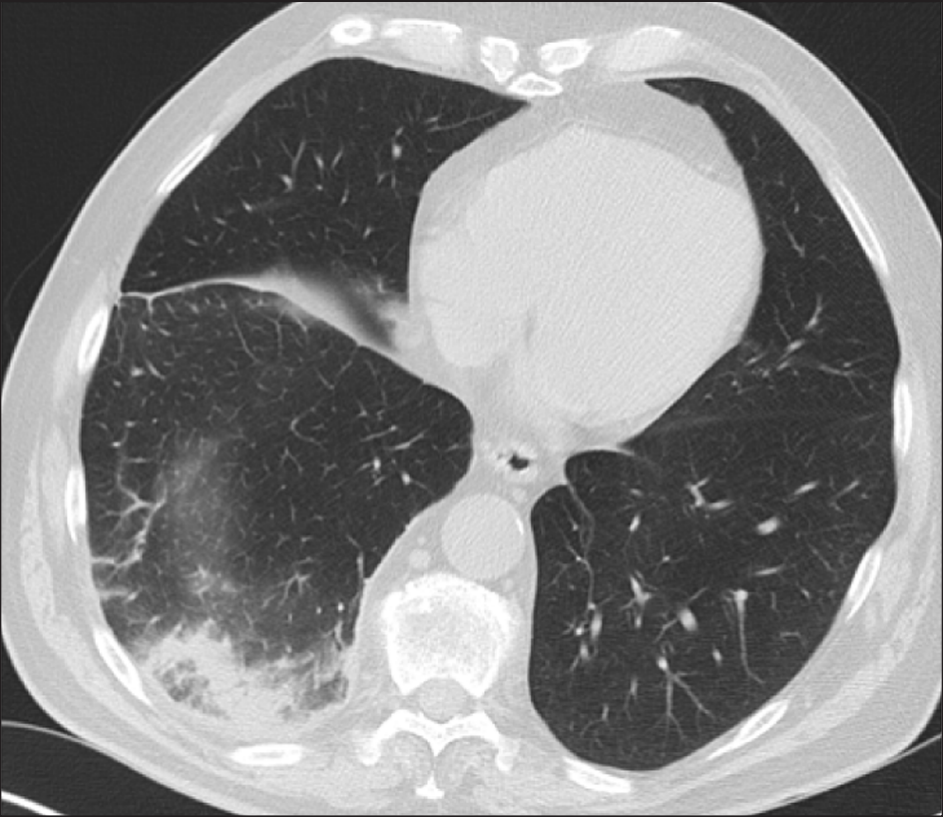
HRCT with a ground-glass opacity surrounded by a crescentic consolidated zone (RHS) in the subpleural space of the RLL.
